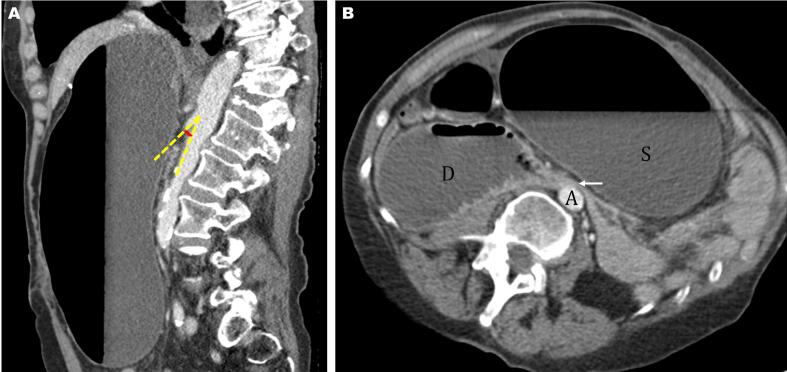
Postoperative abdominal computerized tomography (CT) images: A) Sagittal view contrast-enhanced CT shows a narrow aortomesenteric angle of 16.6 degrees (dashed yellow line) and a reduced aortomesenteric distance of 5.9 mm (red line). B) Axial view contrast-enhanced CT shows dilated stomach (S) and proximal duodenum (D), with transition point (white arrow) located at aortomesenteric level. (For interpretation of the references to colour in this figure legend, the reader is referred to the web version of this article.)
